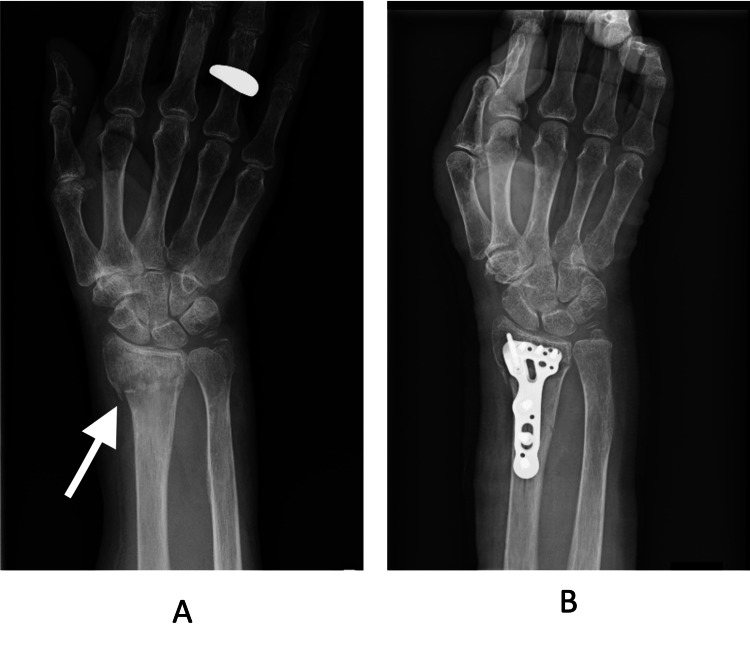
Preoperative and postoperative radiographs of the distal radius fracture. Radiographs of the right wrist in a patient with a distal radius fracture (AO classification A2). (A) Preoperative radiograph. (B) Postoperative radiograph obtained on the day of surgery after open reduction and internal fixation using a volar locking plate.
